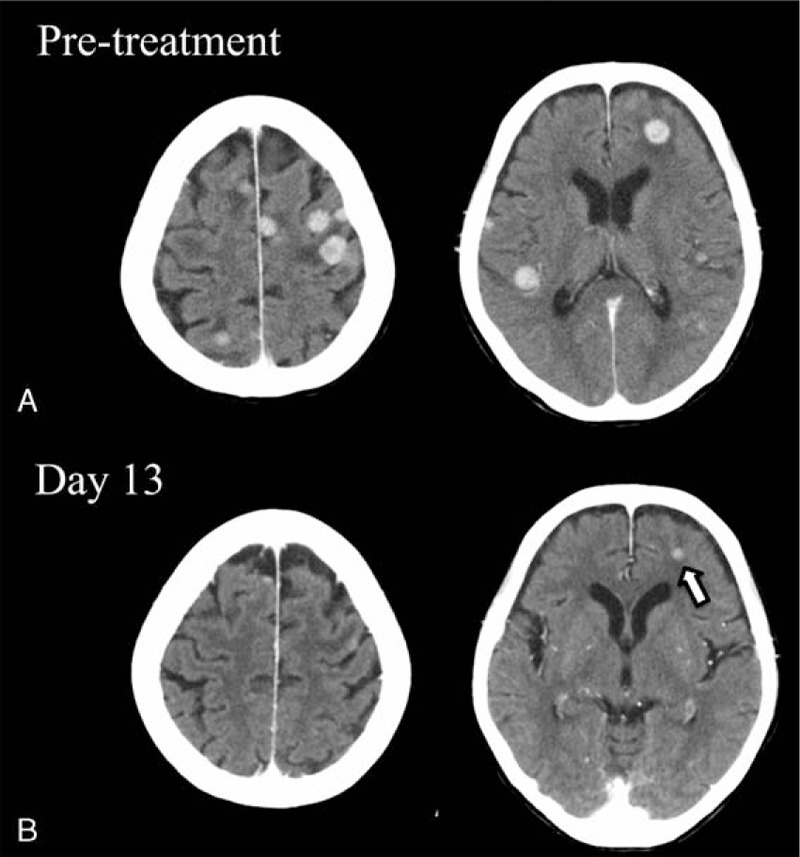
Contrast-enhanced axial brain CT images of case 1. Multiple metastases at baseline. Complete remission of brain metastases except for 1 (white allow) in the left frontal lobe after 13 days osimertinib administration. CT = computed tomography.
